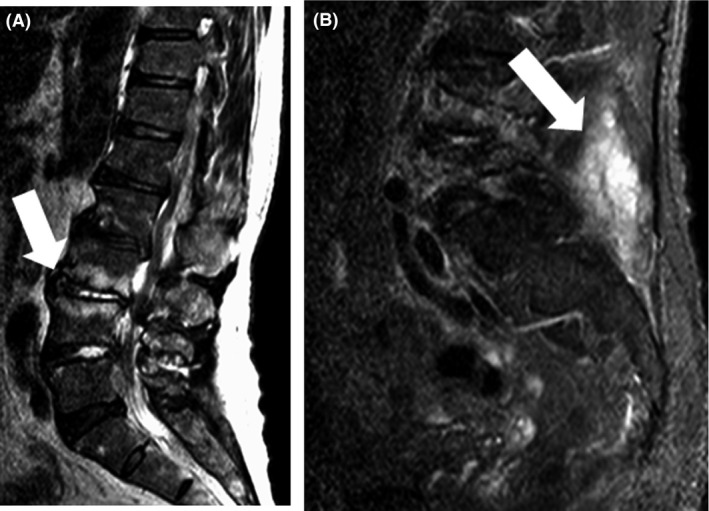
Magnetic resonance imaging on post‐admission day 4 in a 65‐year‐old man admitted with meningeal irritation. A, Pyogenic spondylitis is seen from L1 to S1 (arrows). B, Inflammation in connective tissue is seen in the dorsal lumbar region (arrows).
